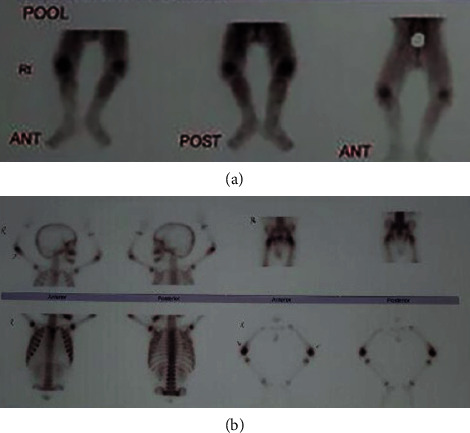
Three-phase bone scintigraphy shows inflammatory processes in several joints, including both left and right knees. (a) Immediate phase and (b) delayed phase (for the first case).
